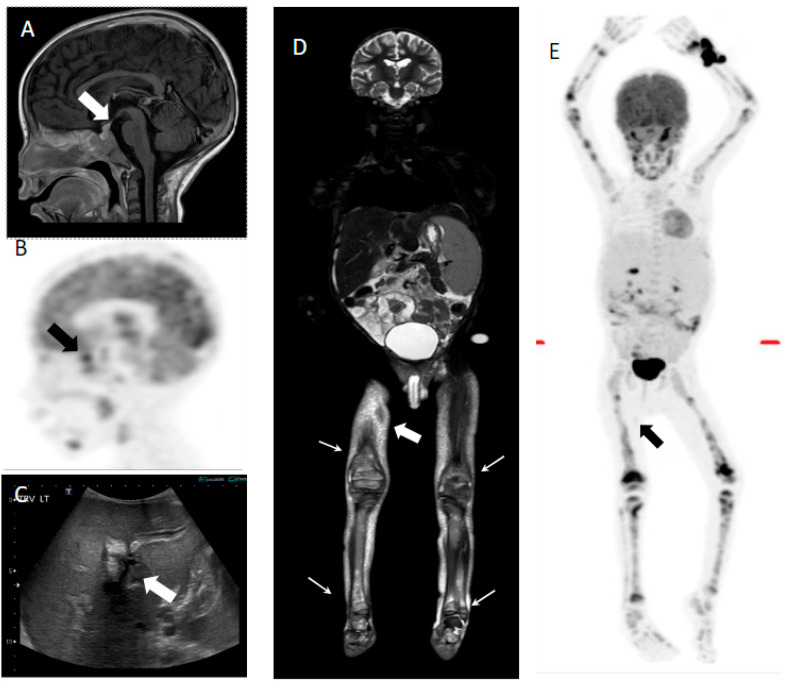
Seven-year-old male with multisystem LCH. (A) Sagittal T1 post-contrast image shows thickening and enhancement of the pituitary stalk (white arrow). (B) FDG PET shows increased uptake in the sellar region (black arrow). (C) Transverse ultrasound image shows hepatomegaly and increased periportal echogenicity/edema (white arrow). (E) Whole-body coronal STIR MRI image shows hepatosplenomegaly, ascites, multifocal areas of increased signal in the bone marrow (white arrows), and cutaneous involvement (white filled arrow). (D) MIP PET image demonstrates multifocal avid FDG disease involving the axial and appendicular skeleton. Mild focus of uptake is seen in the right mid-thigh, corresponding to a subcutaneous lesion (black arrow). Biopsy of the skin lesions was positive for LCH.
